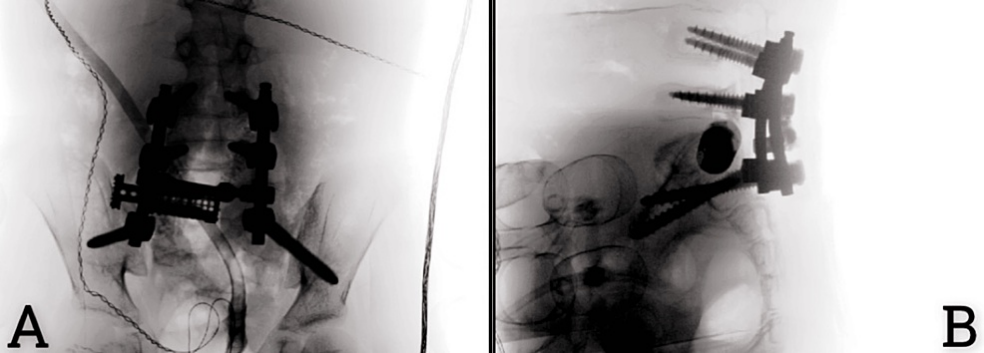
(A) Anteroposterior and (B) lateral lumbosacral X-rays show L4/5 instrumentation with an S1 expandable cage horizontally placed from the S1 body to the sacro-illiac joint on the left side, with bilateral S2 iliac screws containing a horizontally placed cage in S1
